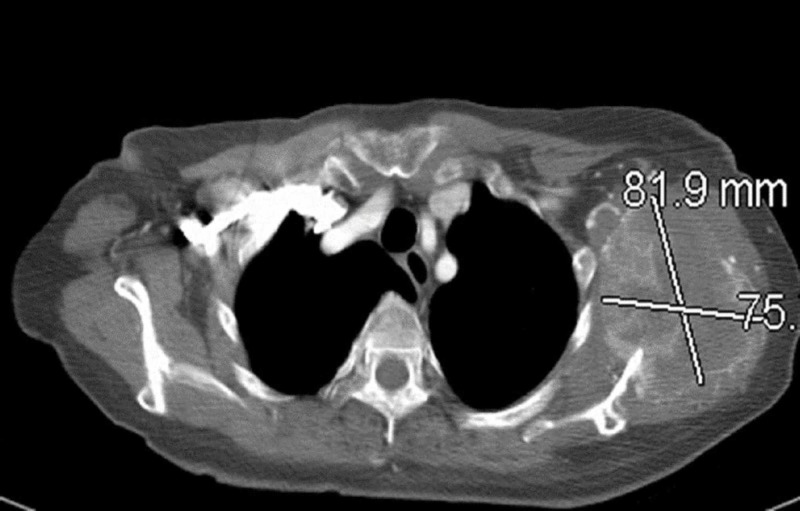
CT chest showing 8.2 cm x 7.6 cm expansile lytic lesion in the left scapula.
